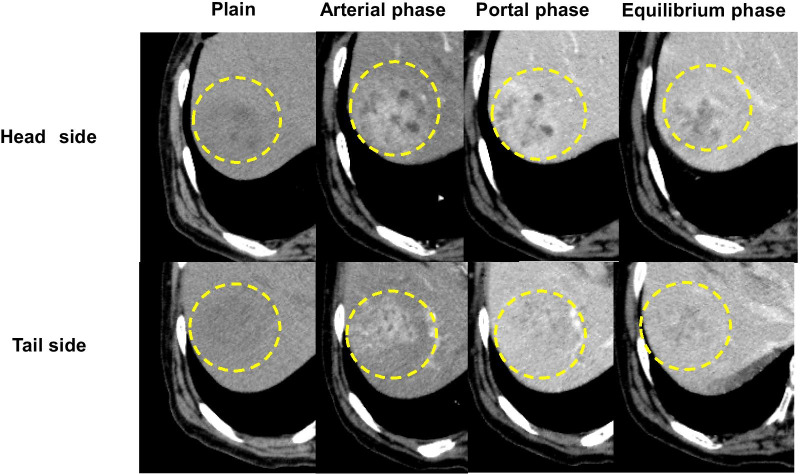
Abdominal contrast-enhanced computed tomography (CT) scan. An abdominal contrast-enhanced CT scan revealed a tumor 3 cm in size in liver segment 8 that showed heterogeneous enhancement in the arterial phase and washed out insufficiently in the portal and equilibrium phase (dotted circle)
